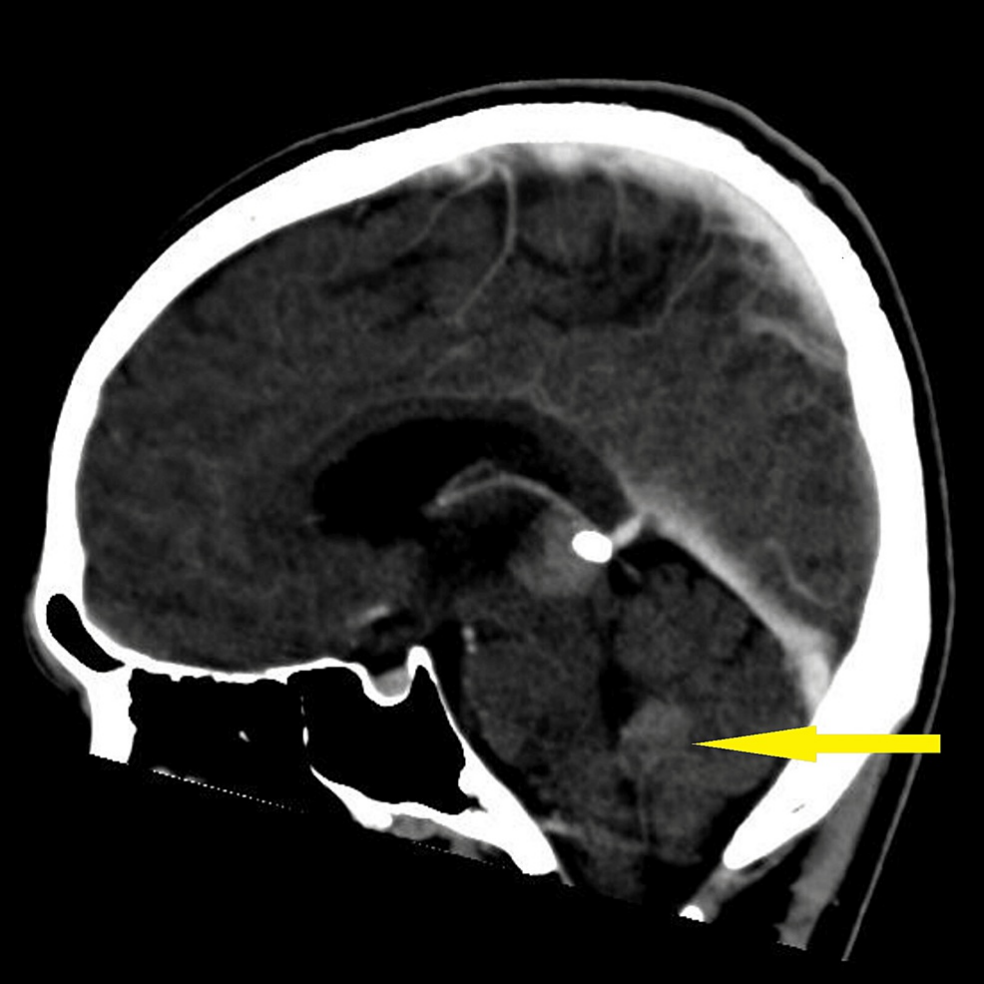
CT brain sagittal showing hypodense lesion in the right lateral and posterior wall of the fourth ventricle measuring 2.1 cm x 3.3 cm x 2.2 cm (yellow arrow)
